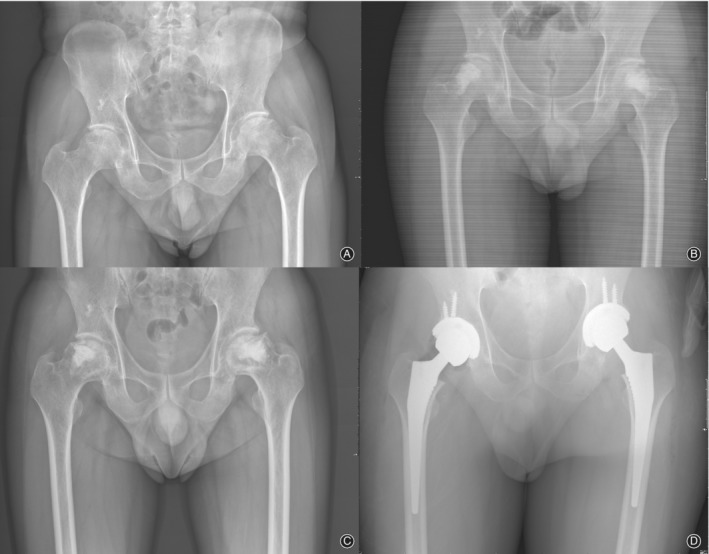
(A) Radiographs of a 36‐year‐old female patient with bilateral avascular necrosis of femoral head. (B) Postoperative radiograph showing that patient was underwent bilateral BIG of the femoral head. (C) Radiographs taken 20 months post operation show a progressive collapse of the bilateral femoral head. (D) Last follow‐up plain radiograph.
